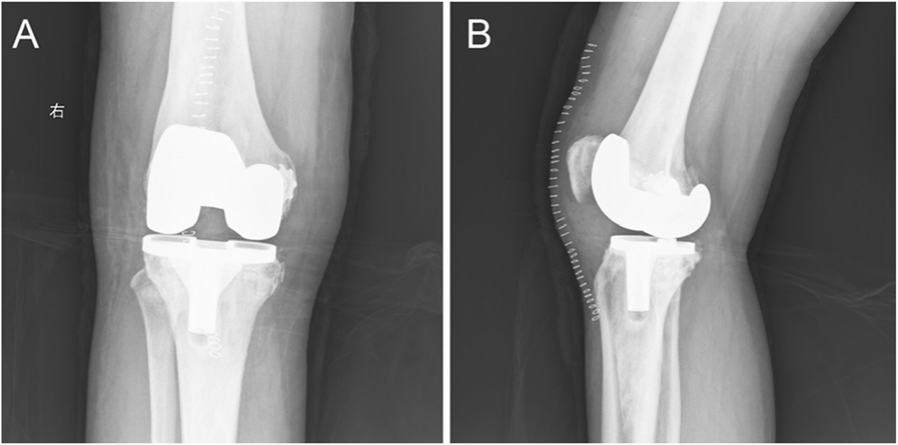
Postoperative radiograph of the right knee. An avulsion fracture occurred on the femoral medial epicondyle. A small spider-like plate was used to fix the fracture
